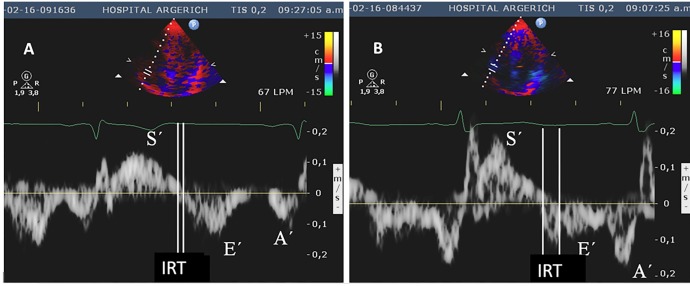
Doppler tissue imaging of the basal portion of the right
